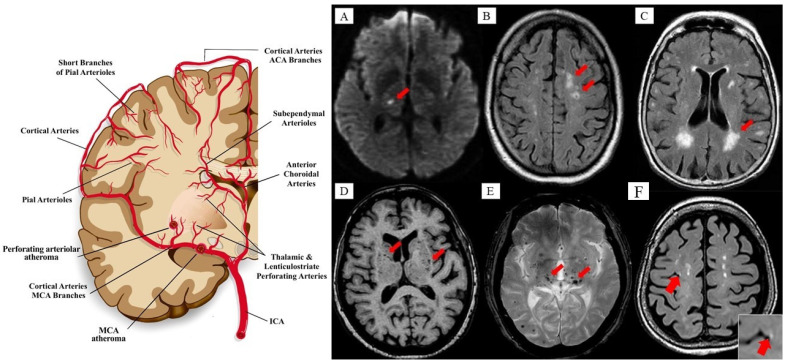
Vascular blood (Penetrating Arterial) supply to the brain, vasculo-pathological process (i.e., atheroma) of cerebral small vessel disease (CSVD), and neuroimaging manifestation and characterization of CSVD based on STandards for Reporting Vascular changes on nEuroimaging (STRIVE). (A) Diffusion-weighted imaging (DWI) showing recent small subcortical infarct (red arrow). Usual diameter is around 3–15 mm, with hyperintense rim surrounding ovoid cavity. It can also be seen as increased signal intensities in fluid-attenuated inverse recovery (FLAIR), T2-weighted, and DWI and decreased T1-weighted signal with isointense in T2*-weighted gradient-recoiled echo (GRE) signal and susceptibility-weighted imaging (SWI). It is best identified through DWI with usual infarct diameter of ≤20 mm. (B) FLAIR showing lacunar infracts (red arrow). Lacunar infarcts appeared as increased hyperintensity in T2-weighted signal, decreased T1-weighted, and FLAIR signal and isointense in DWI. Usual diameter is around 3–15 mm, with hyperintense rim surrounding ovoid cavity. (C) FLAIR showing white matter hyperintensities (WMHs) of presumed vascular origin (red arrow). WMHs seen as increased intensity or hyperintensity on T2-weighted imaging, T2*-weighted GRE and FLAIR (best identified), isointense on DWI, and hypointense (decreased intensity) on T1-weighted imaging. (D) T1-weighted imaging showing enlarged perivascular spaces (ePVS) (red arrow) with usual diameter of ≤2 mm. ePVS is seen as decreased FLAIR and T1-weighted signal intensity, with increased T2-weighted signal. Meanwhile, T2*-weighted GRE and DWI appeared isointense, and they also appeared in similar signal intensity with cerebrospinal fluid (CSF). (E) T2*-GRE showing cerebral microbleeds (CMBs) (red arrow). CMBs are small, rounded areas of signal void with blooming, whereby they were visualized as isointense T1- and T2-weighted signal, FLAIR, and DWI. They are best identified under T2*-weighted GRE or SWI as reduced signal intensities. Usual diameter is around ≤10 mm (mostly 2–5 mm). (F) T1-weighted (hypointense) of 3T MRI showing cortical microinfarcts (red arrow). Images (A–E), reproduced from [105], Frontiers, 2019, image F was adapted from [106], Mayo Clinic, 2019. ACA, anterior cerebral arteries; ICA, internal carotid artery; MCA, middle cerebral arteries.
